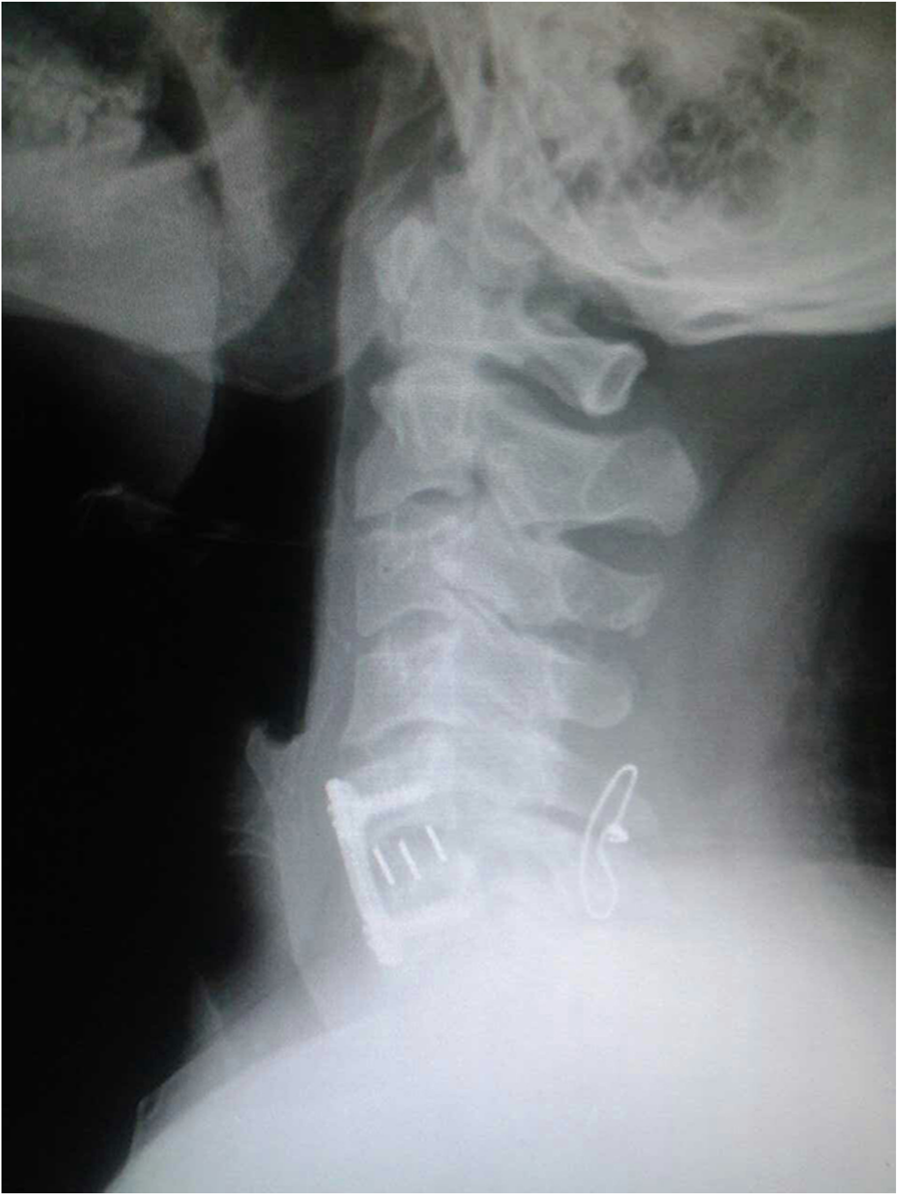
Postoperative C-spine anteroposterior radiograph: anterior fusion and posterior fixation with wire
